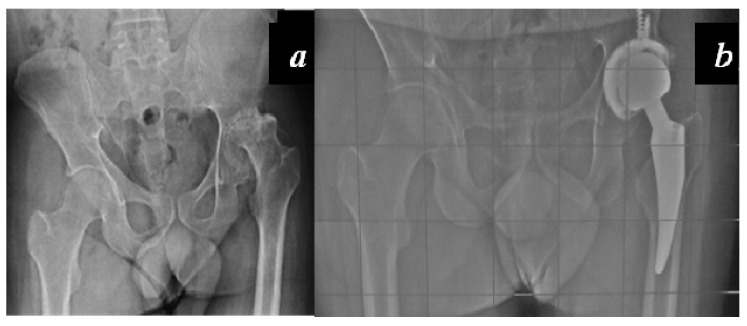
(a) Preoperative x-rays of the left hip of a 33-year-old patient affected by severe haemophilia A; (b) X-rays 3 years after surgery: cementless short stem, acetabular cup fixed with two supplementary screws and COP coupling.
